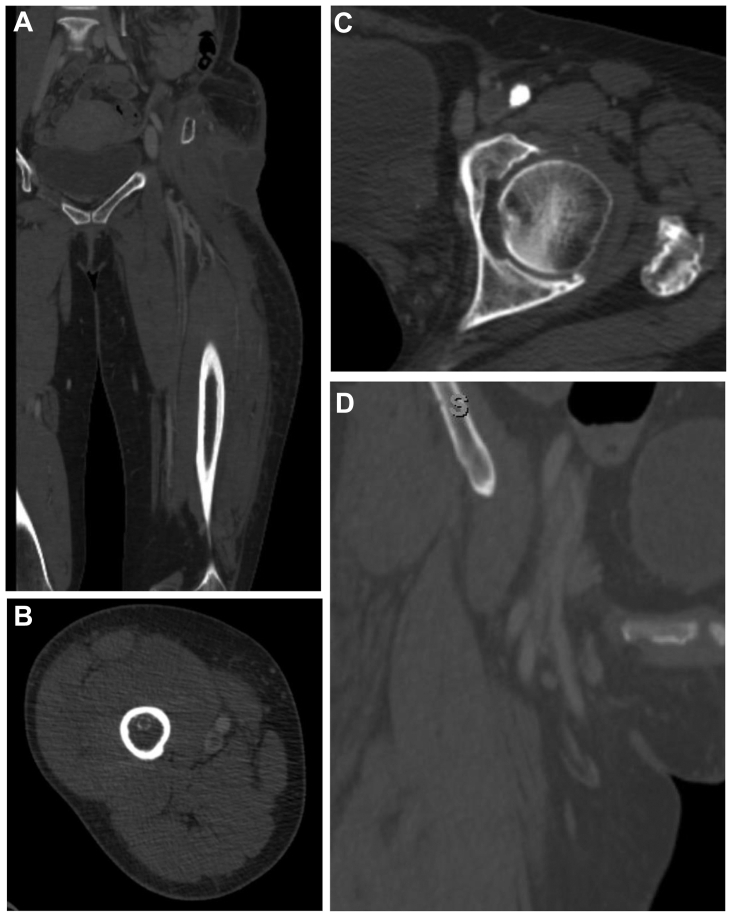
Representative images from CT scans positive for the diagnosis of DVT from four patients: (A) patient 77; (B) patient 85; (C) patient 23; (D) patient 53. *CT*, Computed tomography; *DVT*, deep venous thrombosis.
